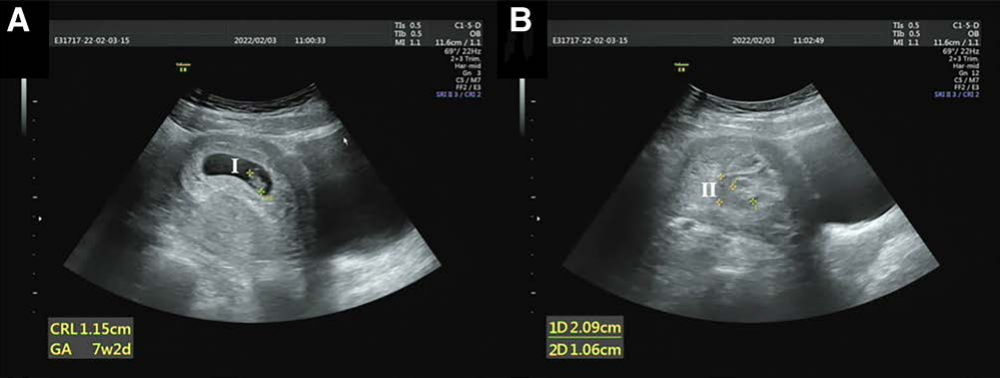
Transabdominal ultrasonography on postoperative day 7. (A) The intrauterine pregnancy sac, yolk sac, fetal bud, and fetal heartbeat are visible (I); the length of the fetal bud is approximately 1.8 cm. (B) A dark fluid area of approximately 2.6 × 1.2 × 2.2 cm is detected in the lower uterine cavity below the pregnancy sac with poor internal sound penetration (II). No abnormal echo is found in the cervix.
